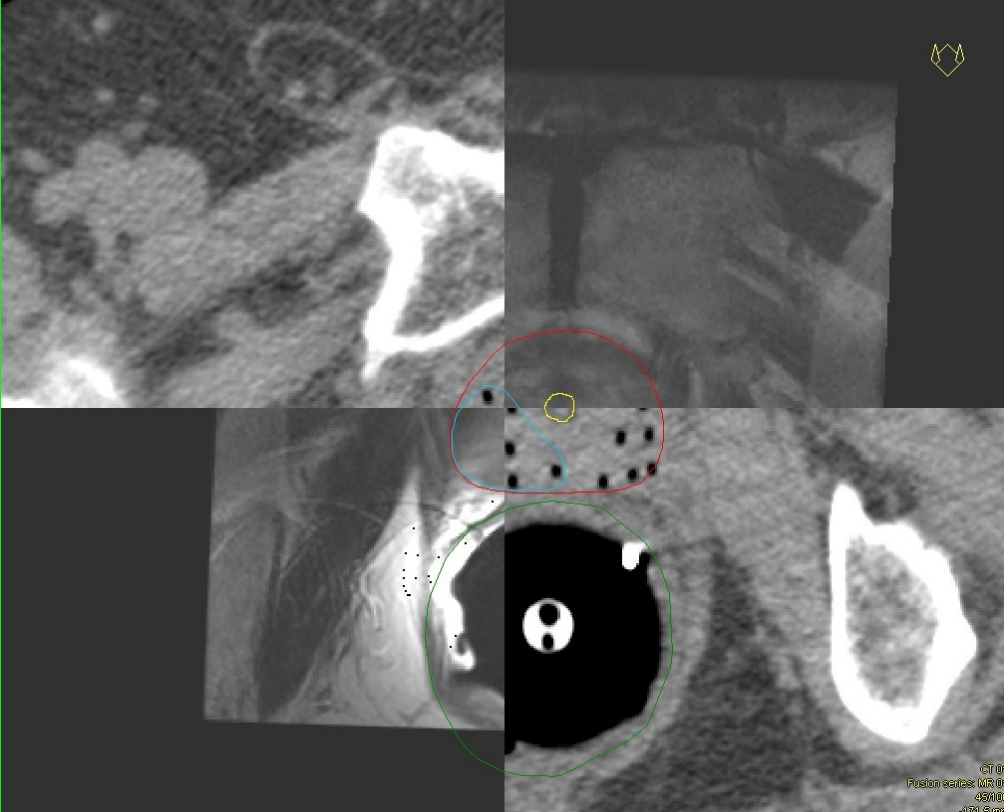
Image fusion between the MRI and the post-implantation CT with catheters to delineate the DIL to boost
